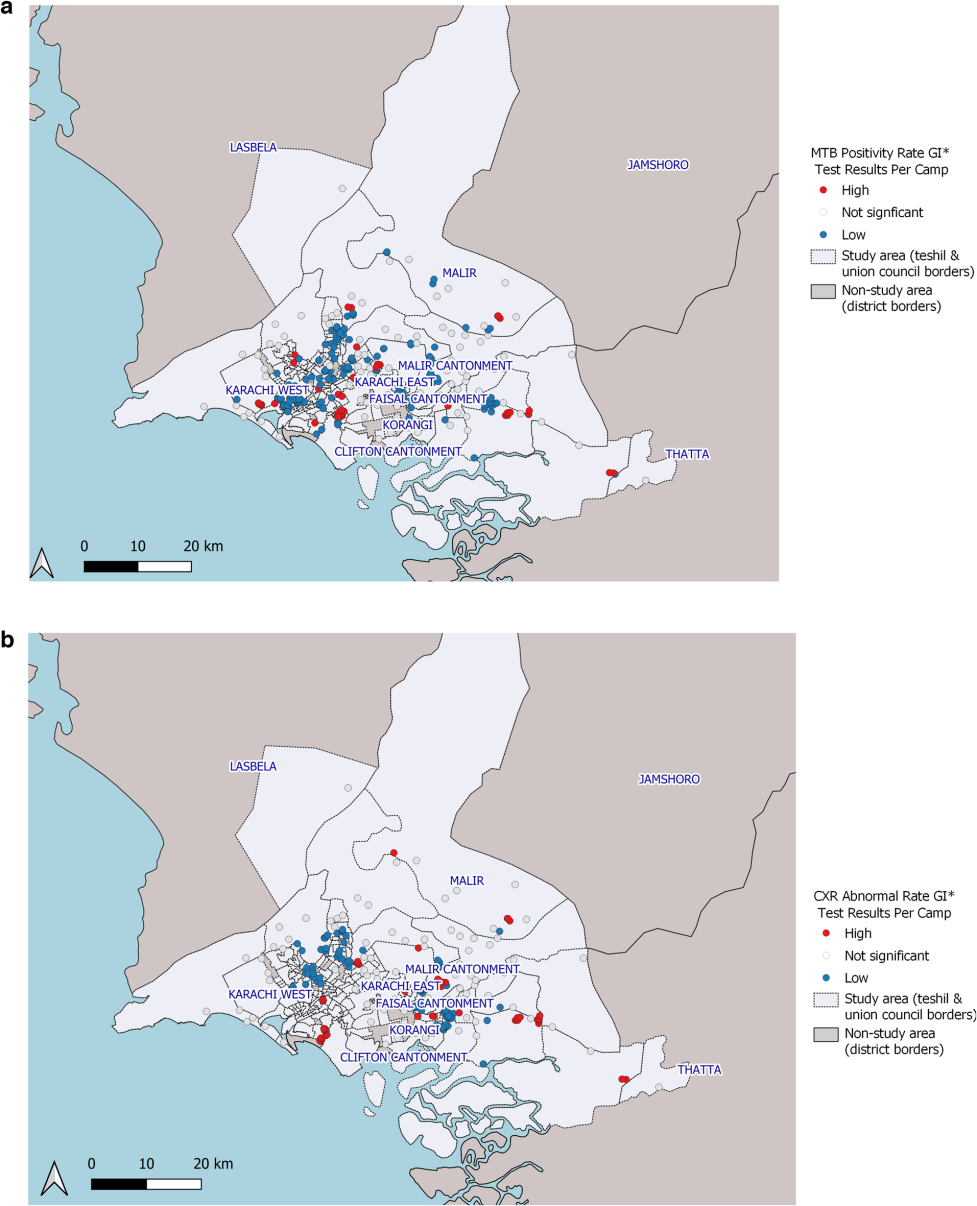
(a) GI* analysis (distance-based method) for MTB positivity rates from locations of mobile chest X-ray supported active case-finding camps in Karachi, Pakistan (July 2018–March 2020). A high–high result indicates clustering of GPS locations of camps with high MTB positivity. A low–low result indicates clustering of GPS locations of camps with low MTB positivity. QGIS Geographic Information System v3.26.3. QGIS.org, 2022. QGIS Association. http://www.qgis.org. (b) GI* analysis (distance-based method) for abnormal X-rays rates from locations of mobile chest X-ray supported active case-finding camps in Karachi, Pakistan (July 2018–March 2020). A high–high result indicates clustering of GPS locations of camps with high abnormal X-ray rates. A low–low result indicates clustering of GPS locations of camps with low abnormal X-ray rates. QGIS Geographic Information System v3.26.3. QGIS.org, 2022. QGIS Association. http://www.qgis.org.
